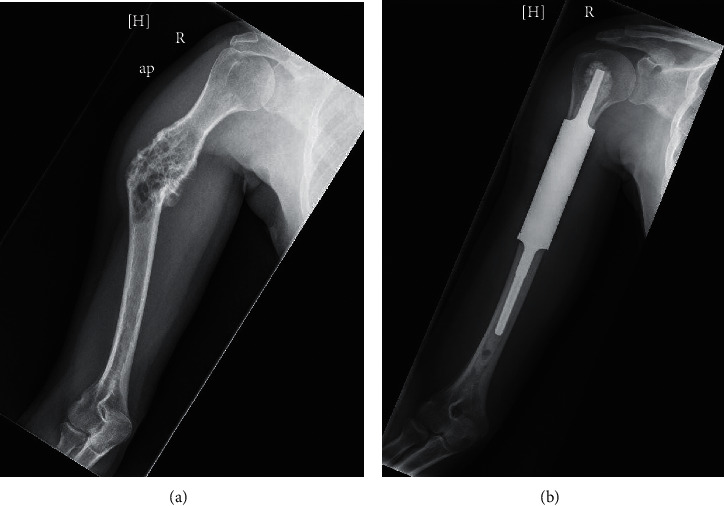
(A) Preoperative radiograph showing lytic lesion in the humerus shaft with deformity. (b) Postoperative radiograph showing well fixed intercalary humerus prosthesis.
